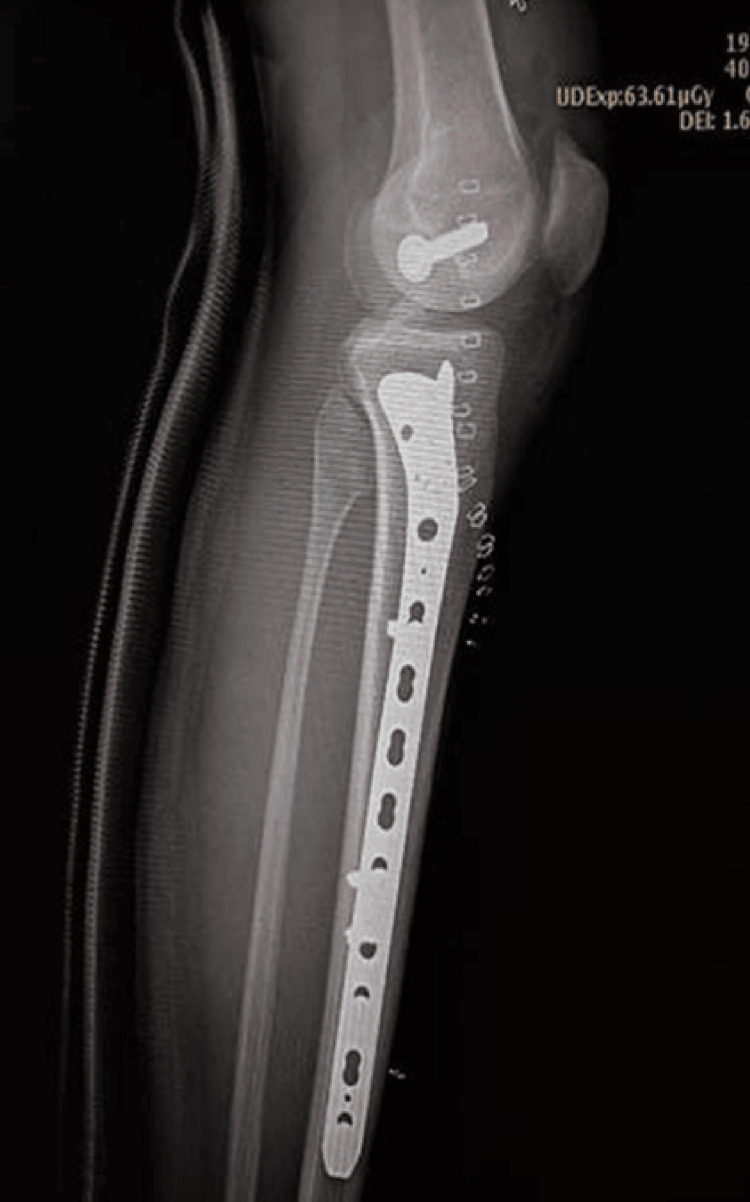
Immediate Postoperative Radiograph of the Left Lower Limb Showing Fraser Type II-C Floating Knee Injury (Closed Type) Following Surgical Fixation.
